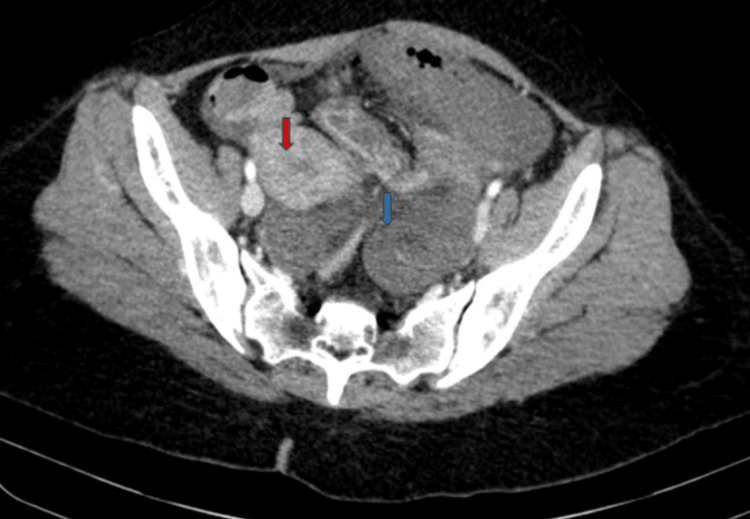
Computed tomography showing ischemic small bowel loops. In the axial plane computed tomography, there was evident diffuse circumferential thickening of distal ileal loops and suboptimal mucosal enhancement (blue arrow), all localized in the pelvic region. Red arrow showing anterior and right lateral displacement of the uterus.
